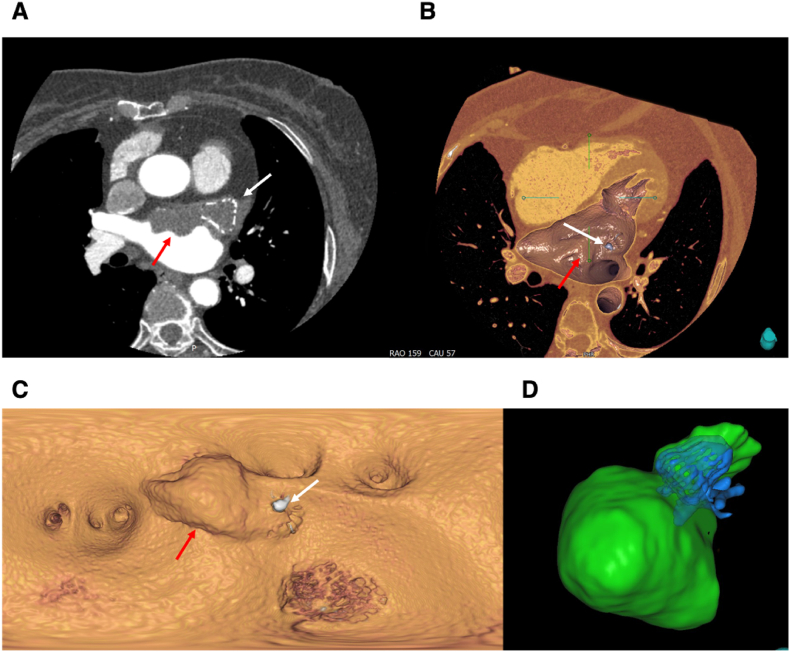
First Follow up Computed Tomography Fifty Days After Implantation Demonstrating Device Related Thrombosis (A) Axial cross-sectional CT image showing the Watchman FLX occlude (white arrow) and adjacent atrial surface thrombus (red arrow). (B) Three-dimensional volume-rendered reconstruction highlighting the occluder (white arrow) and overlying thrombus (red arrow). (C) Virtual endoscopic view revealing a hypoattenuating layered thrombus (red arrows) adherent to the atrial surface of the device (white arrow), consistent with early DRT. (D) Volume-rendered reconstruction demonstrating the Watchman FLX well seated within the LAA without displacement or significant peridevice leak, indicating that thrombus formation occurred despite optimal device placement. (For interpretation of the references to colour in this figure legend, the reader is referred to the Web version of this article.)
